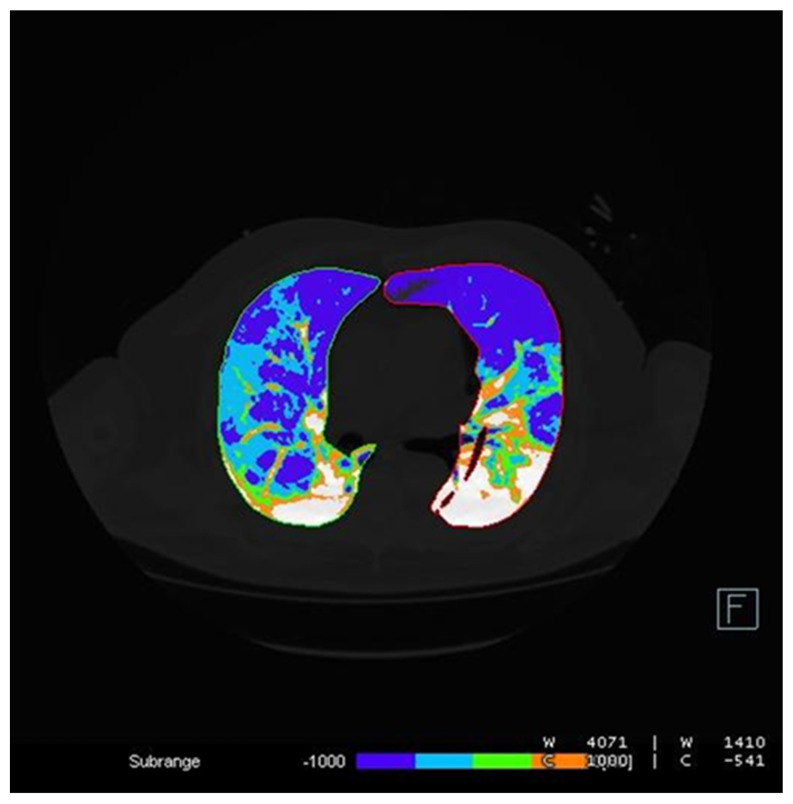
CT Pulmo 3D: lung contouring. This figure shows the lung parenchyma lesions counted; the different colors employed suggest different HU sub-ranges.
